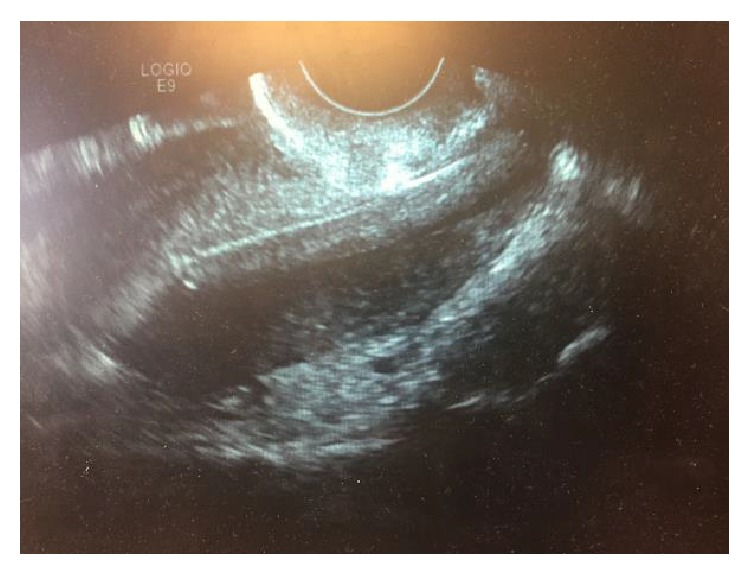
Transvaginal ultrasonography demonstrating retained luminaria.
